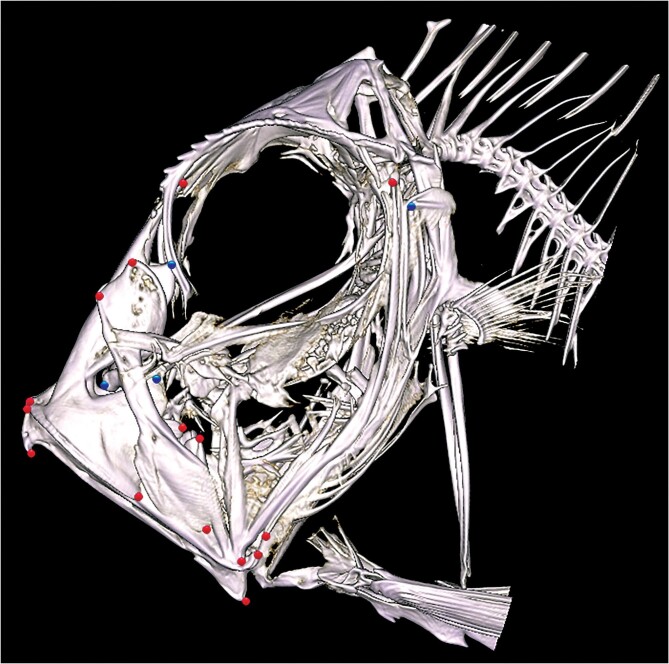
2-D image in lateral view of an isosurface µ-CT reconstruction (Fedorov et al. 2012) of *C. rosea* (FMNH 67,093), showing the external (red) and internal (blue) landmarks.
